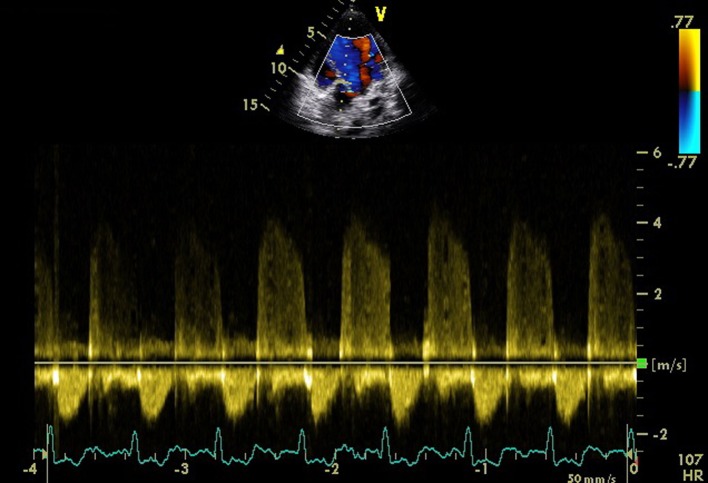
Color Doppler in apical five-chamber view showed mild aortic regurgitation.
